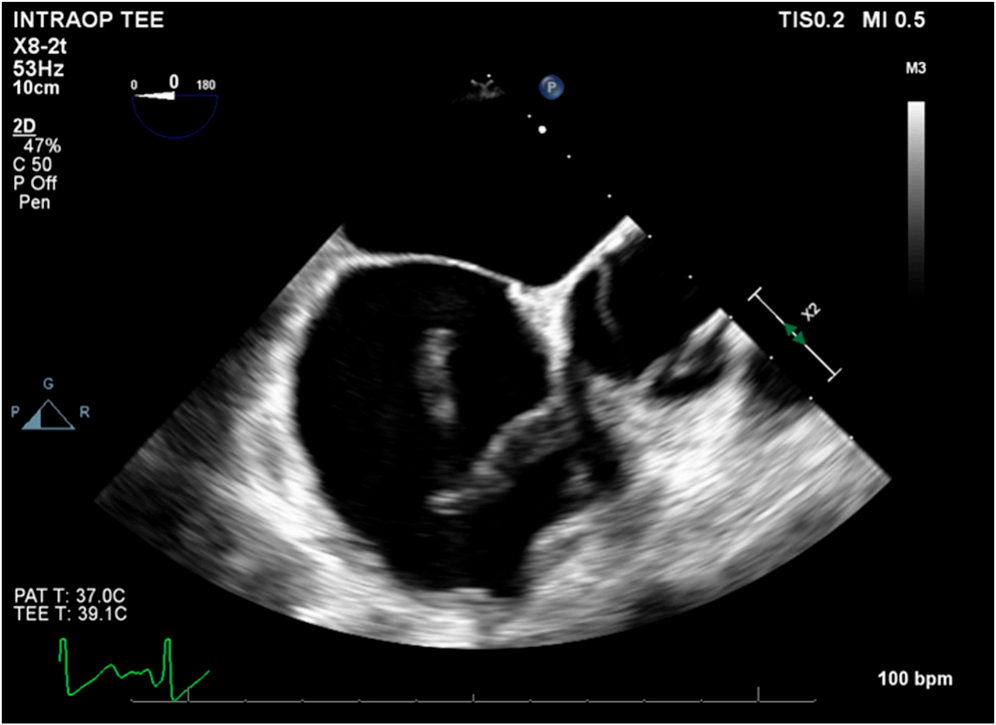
Intraoperative transesophageal echocardiogram with demonstration of atrial thrombus.
